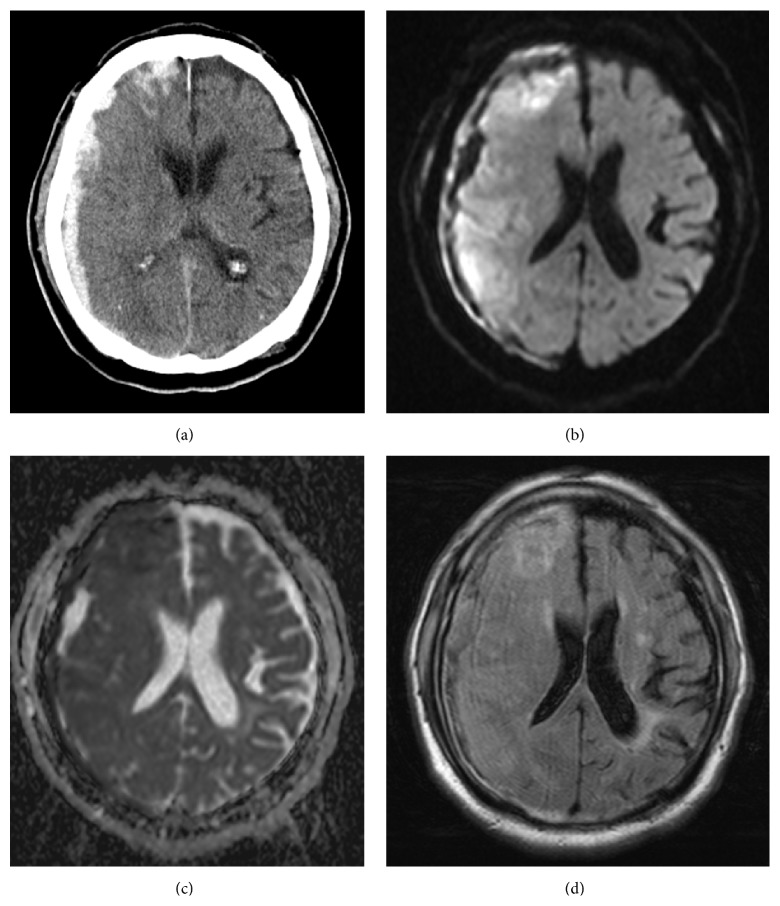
Computed tomography (CT) and magnetic resonance image (MRI) on admission. Brain CT shows a right hemispheric subdural hematoma (a). Diffusion-weighted image of brain MRI shows high signal intensity in frontal and temporal lobes (b). Apparent diffusion coefficient map is low (c) and fluid attenuated inversion recovery shows high signal intensity (d) in the corresponding areas.
